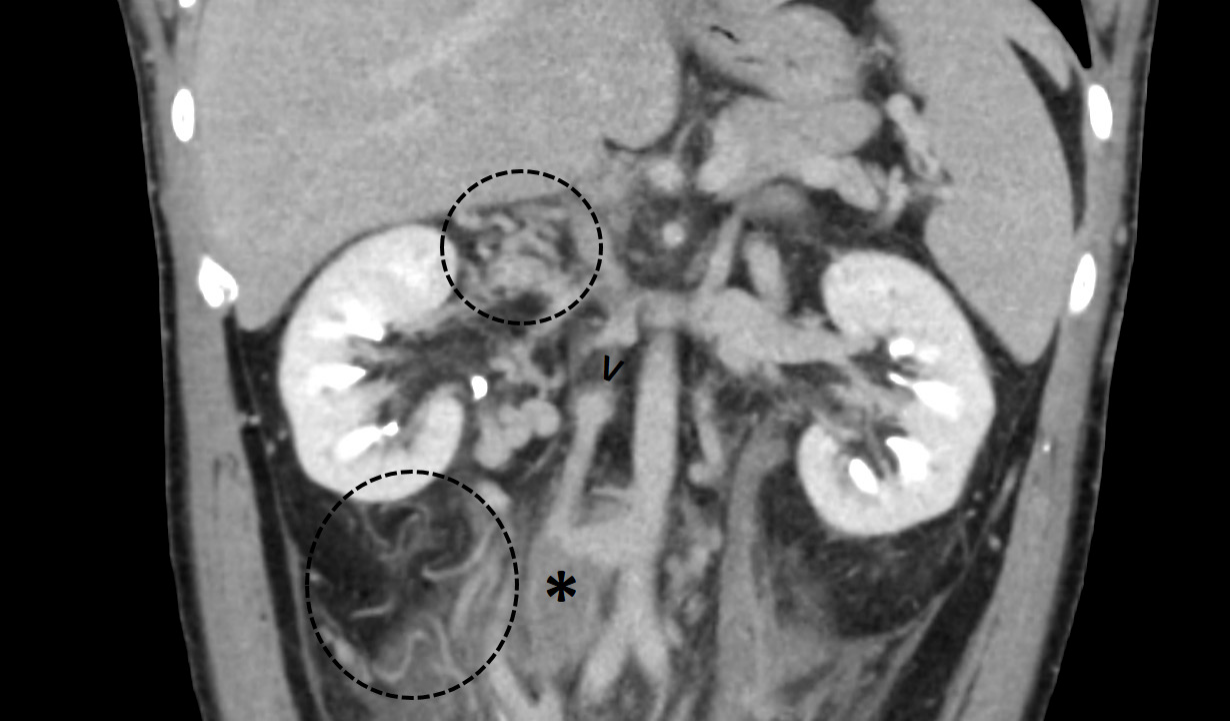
MIP coronal reconstruction of a contrast-enhanced CT, demonstrating the IVC interruption (arrowhead), thrombus in the IVC (asterisk), and multiple collateral veins in the retroperitoneum (dashed circles). IVC, inferior vena cava; MIP, maximum intensity projection.
